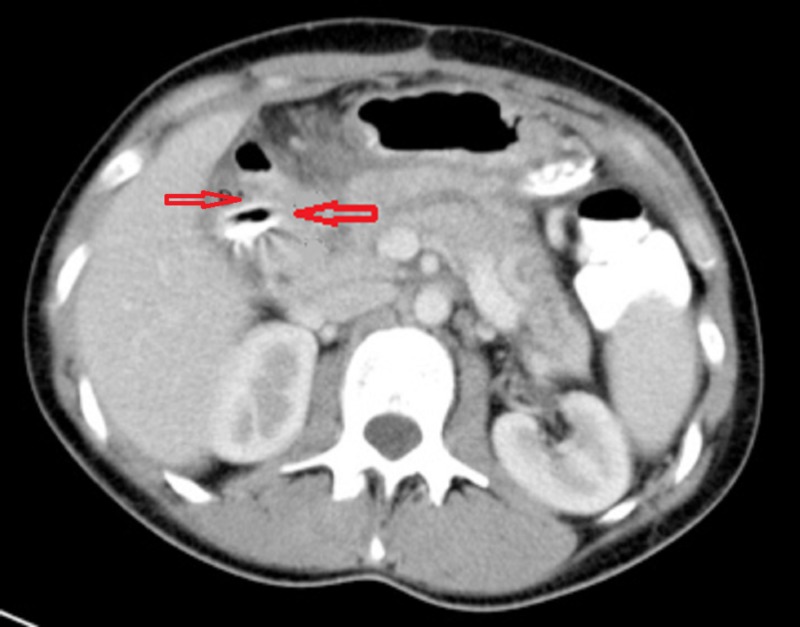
Repeat computed tomography (CT) scan demonstrating migration of lesser sac drain into duodenum. Upper arrow indicates duodenum. Lower arrow shows the path of drain.
